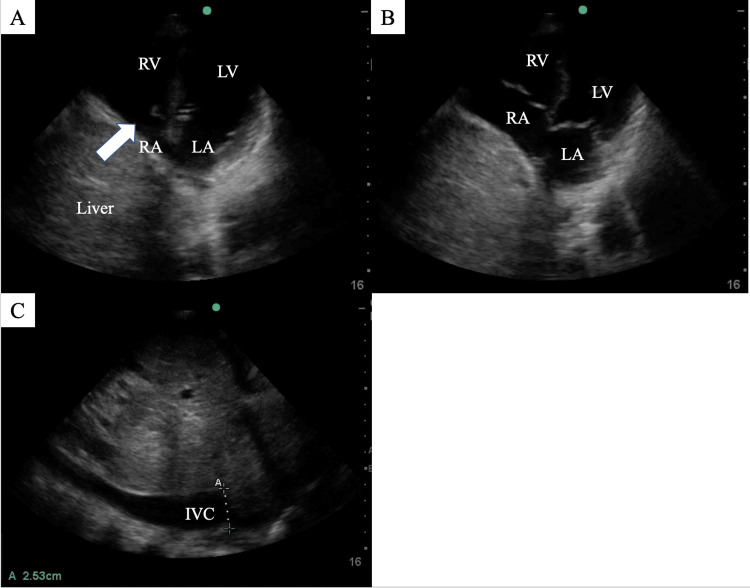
(A, B) Apical four-chamber view: The collapsed right atrium (RA) (a white arrow) compressed by the liver in the patient. (C) The patient’s dilated inferior vena cava (IVC). LA: left atrium; LV: left ventricle; RV: right ventricle
